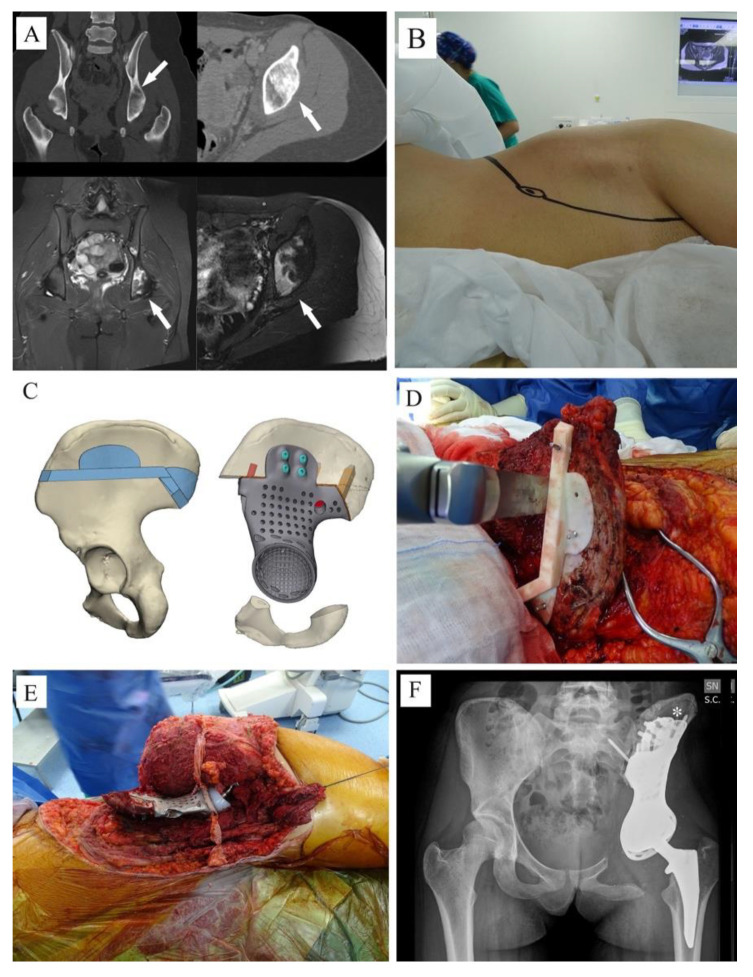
(A) CT-scan and MRI showing a Ewing sarcoma involving the acetabulum (white arrows); (B) due to ischiatic notch involvement, the young patient was positioned in lateral position and an ileo-inguinal approach was performed; (C,D) the tumor was removed using cutting guides; (E): an intraoperative picture showing the 3DPTCMP used to reconstruct the hemipelvis and a neck-sparing short press-fit stem prosthesis used to restore the proximal femur; (F) postoperative X-ray showing the “wafer fixation” (white asterisk); no connection to the residual symphysis or to the ischium was adopted.
